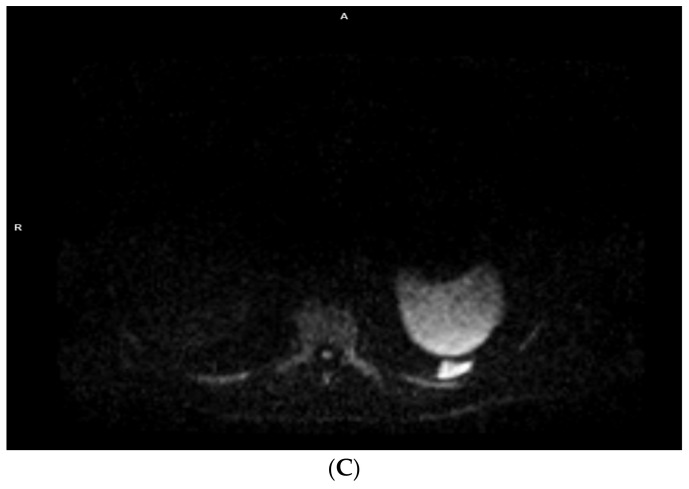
Images of a 70-year-old male patient with colorectal cancer. Focal lesion (meta) adjacent to the dorsal pleura at the base of the left lung. T2-weighted (A), TIRM (B), and DWI b = 800 (C) axial images.
